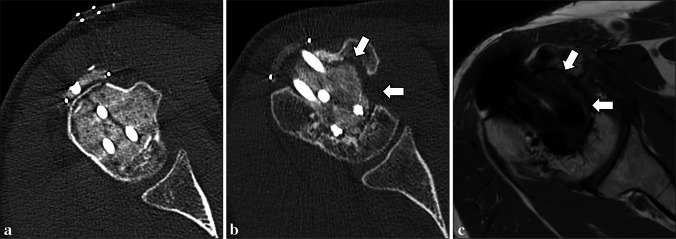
Incomplete bone graft integration in a 54-year-old man. After curettage of an ACT of the proximal humerus, the cavity was entirely filled with bone graft on the CT scan performed on the day of surgery (a). Failure of graft integration occurred along with resorption of the adjacent host bone (arrows), as shown on CT (b) and T1-weighted MRI (c) images at 2-year follow-up
